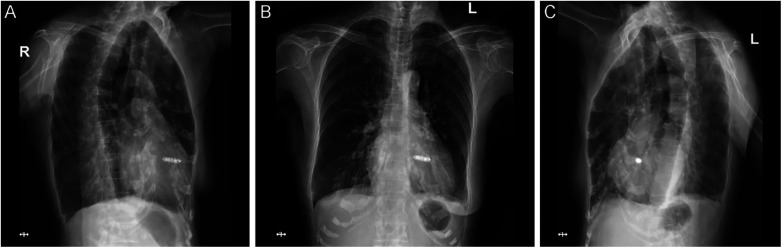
Postoperative chest x-ray at 1-month follow-up showing the stable position of the leadless pacemaker. (A) Right anterior oblique view. (B) Posteroanterior view. (C) Left anterior oblique view.
